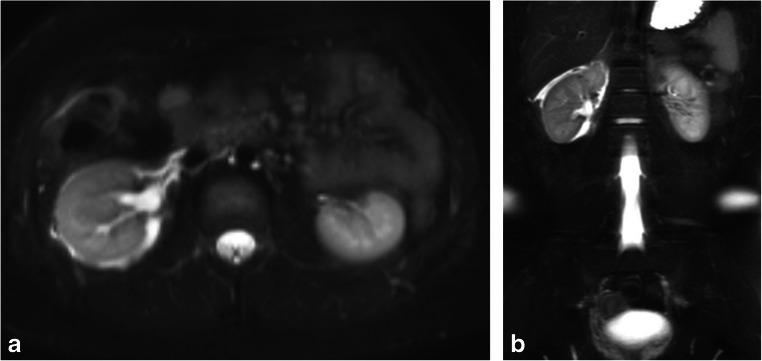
A 15-year-old boy with an alternative diagnosis of obstructing ureterolithiasis. a, b Axial (a) and coronal (b) fat-suppressed T2-weighted single-shot fast spin echo images demonstrate dilatation of the right renal collecting system and enlargement of the right kidney with respect to the left. There is right perinephric edema. This was a discrepant case in which the alternative diagnosis was missed by the interpreting resident. The appendix was normal
